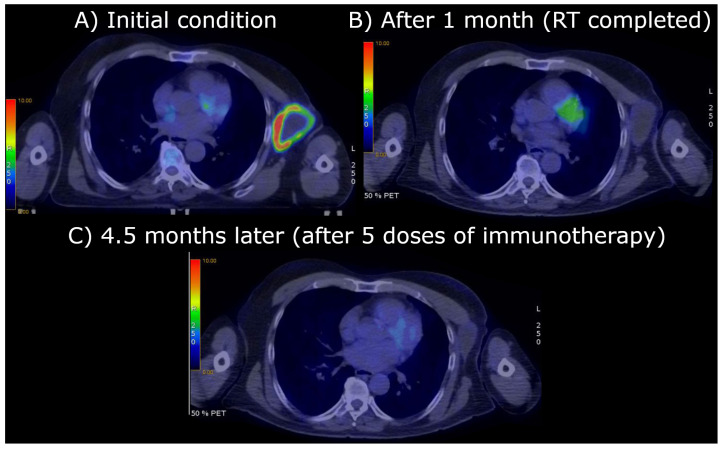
^18^F-FDG PET at different time intervals showing the biggest metastasis located at left axilla: (A) starting condition (171.3 cm^3^); (B) after 1 month and the end of radiotherapy; (C) after 4.5 months and 15 weeks of immunotherapy with cemiplimab (5 intravenous injections).
